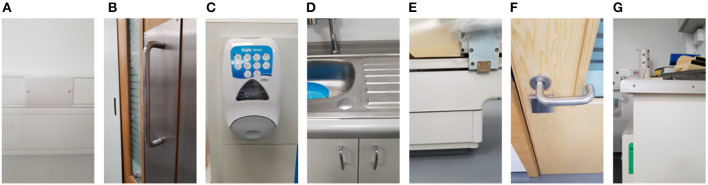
Commonly touched surfaces (A–G) chosen for swab testing in CT scan room of hospital 2.
